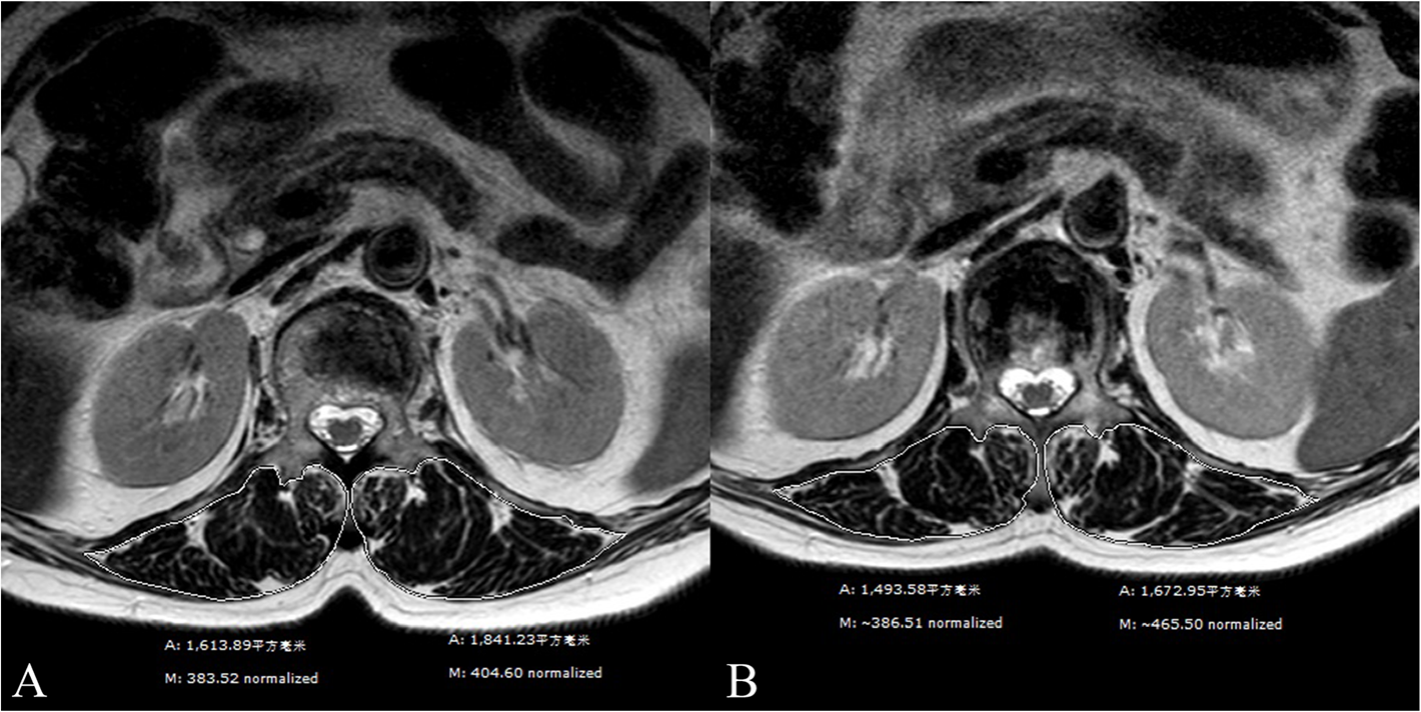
A 76-year-old woman with a OVCF at L1. A Preoperative axial T2-weighted image of L1. B Same image at L1 when T11 fractured after 7 months. The areas of paraspinal muscle decreased significantly. *A* areas, *M* mean grayscale
